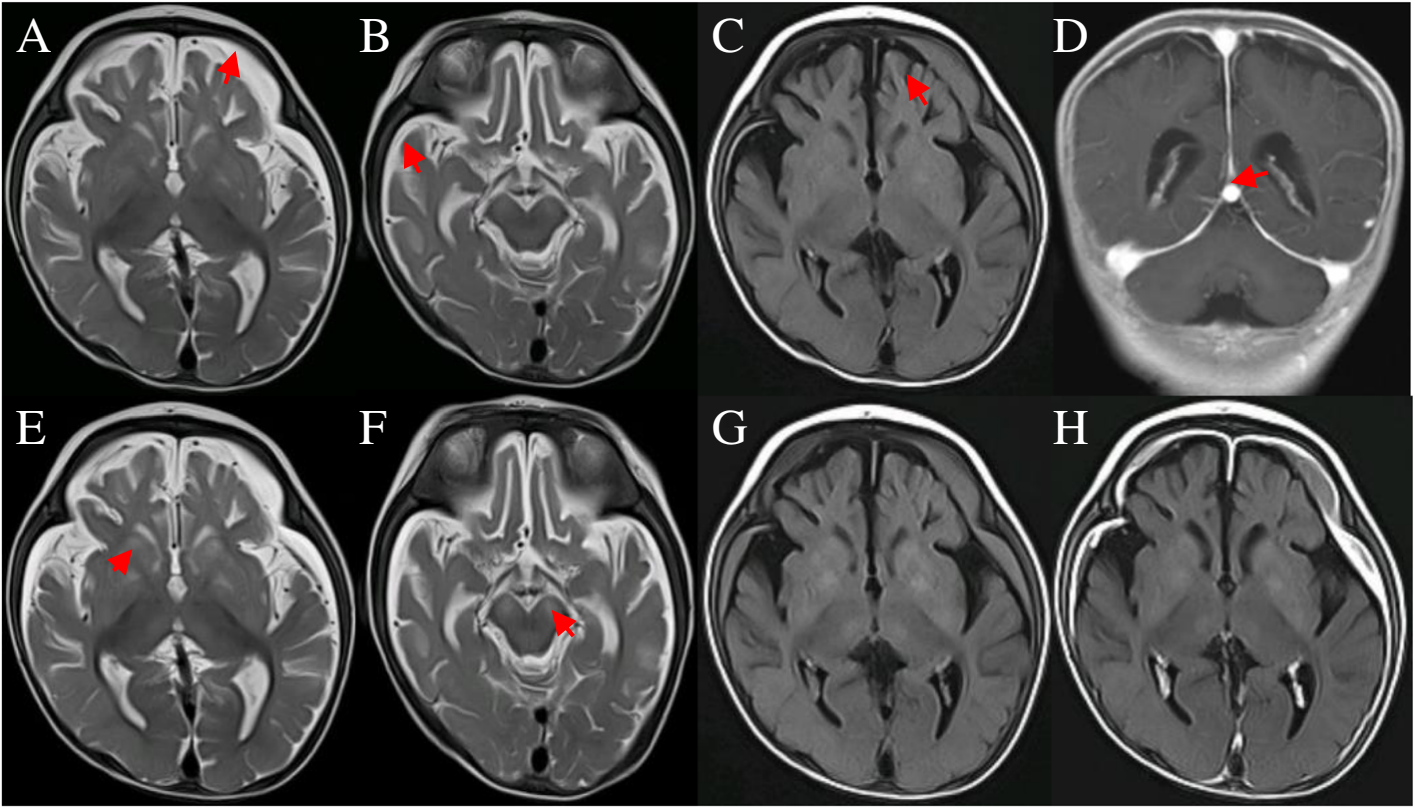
3T Brain MRI of the patient: a to d were performed when he was first transfer to our hospital at 4-month-old. a and b: axial T2WI showed arc-shaped subdural empyema was seen in bilateral frontotemporal parietal lobes. c: axial T2 Flair showed arc-shaped subdural empyema and bilateral cerebral hemisphere atrophy. d: Coronal T1WI enhanced scanning showed bilateral frontotemporal parietal dura enhanced which was called Mercedes-Benz sign. e to h was reexamined 7 days after hospitalization. e and f: axial T2WI showed new symmetrical hypersignal lesions in bilateral basal ganglia, thalamus and cerebral peduncle. g: the lesions had a slightly higher signal on T2Flair. h: the lesions had no obvious enhancement on T2Flair
